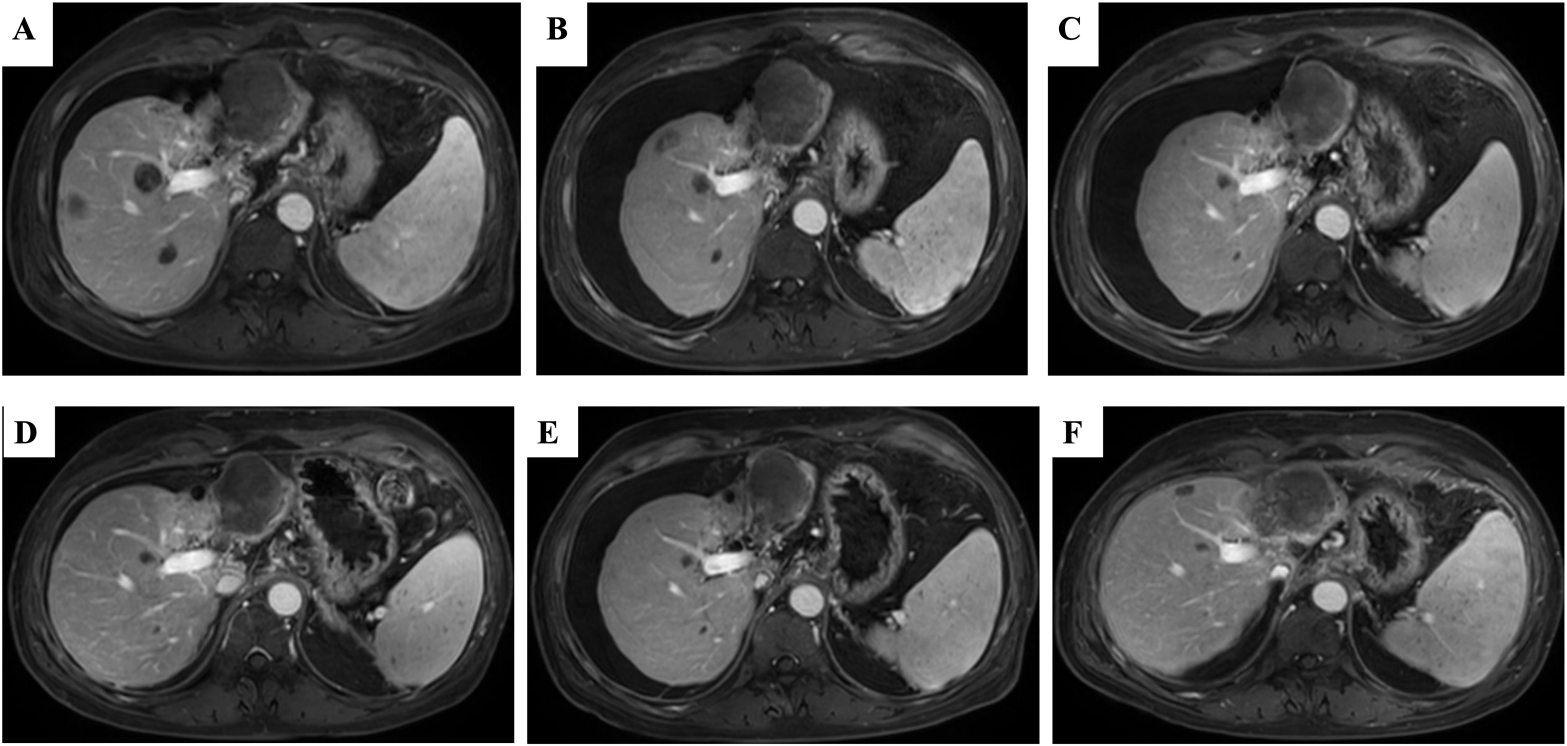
The liver MRI after lenalidomide interruption followed by the initiating of anti-PD-1 treatment and Lenvatinib again. (A) The remains of tumor regression after 3 weeks of Camrelizumab plus Lenvatinib. (B) The remains of tumor were continuous regressed after 3 months of Camrelizumab plus Lenvatinib. (C) The lesions of liver nearly disappeared after 6 months of the combination of lenalidomide and Lenvatinib. (D–F) The disease is still responding until now so far. MRI, magnetic resonance imaging.
